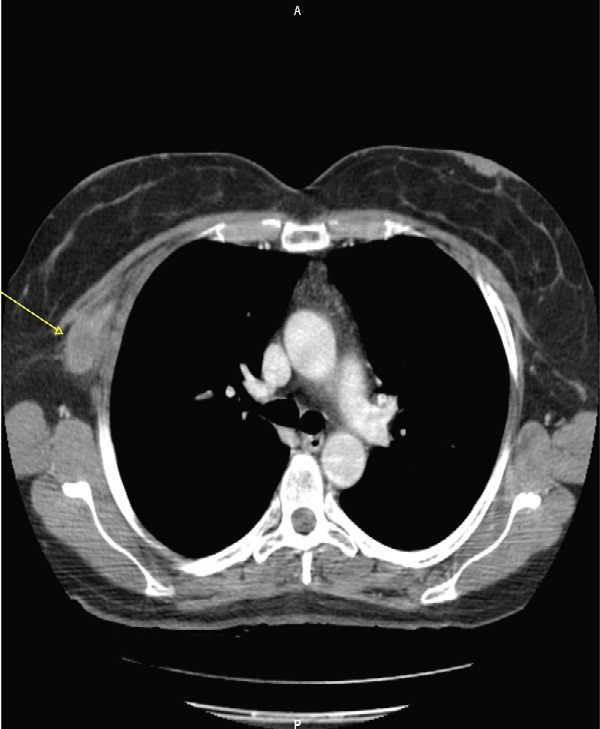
The axial CT scan at first diagnosis, showing a contrast-enhanced solid mass (arrow) in the right axilla, with a total extrapleural growth.
